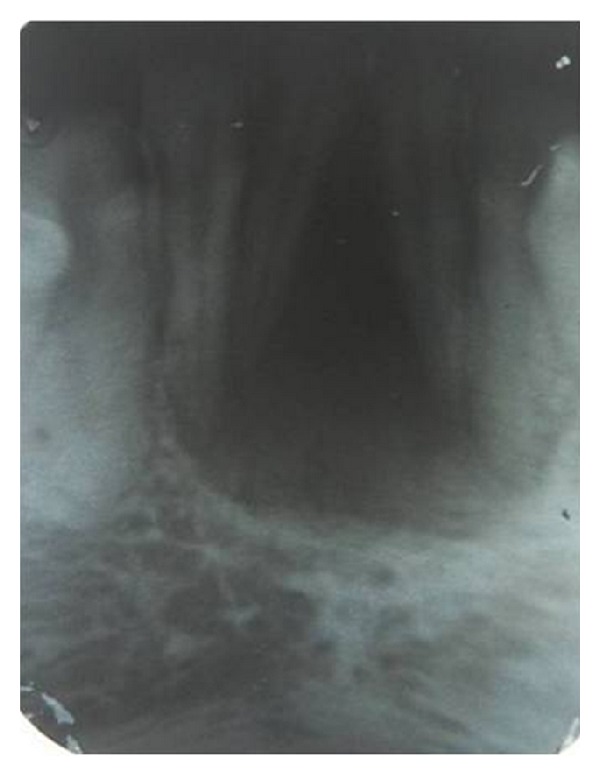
Intraoral periapical radiograph reveals triangular radiolucent area involving roots of mandibular central incisors.
